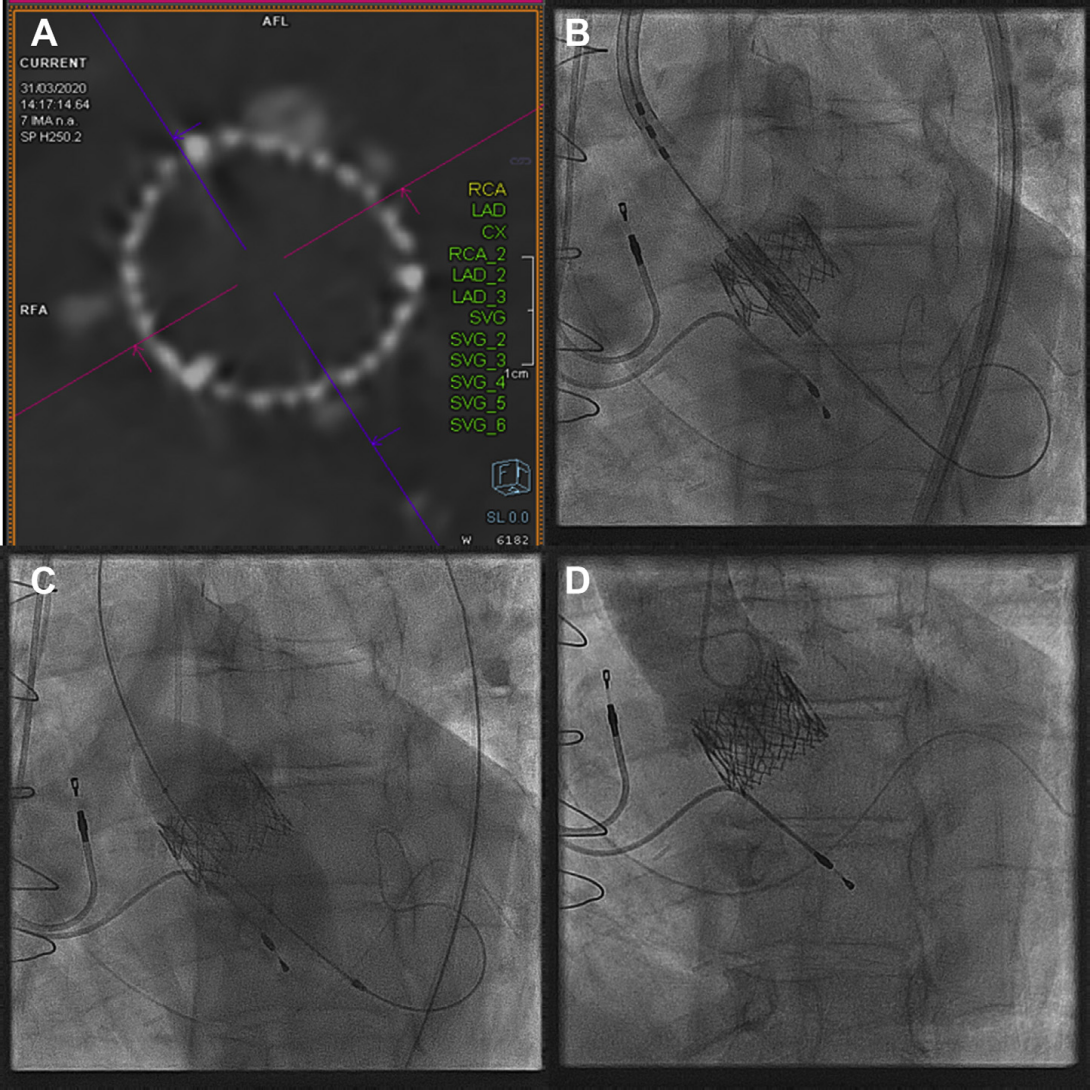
(A) Gated computed tomography aortogram (March 2020) showed complete absence of leaflet calcification of the Sapien XT (Edwards Lifesciences Corp, Irvine, CA) prosthesis (short axis left image, midframe level). (B) In April 2020 redo transcatheter aortic valve replacement was undertaken with a 26 mm Sapien 3 Ultra valve (Edwards Lifesciences Corp) via percutaneous transfemoral access. The top of the Sapien 3 Ultra was aligned with the top of the Sapien XT transcatheter heart valve. (C) Post dilatation was undertaken with a 26-mm Atlas Gold PTA Dilatation Catheter (Bard Peripheral Vascular, Inc, Tempe, AZ) balloon to 12 atm (nominal pressure 4 atm) to optimize expansion of the Sapien 3 and improve valve performance. (D) Root aortography after deployment and after optimization of the 26-mm Sapien 3 Ultra valve showed no aortic regurgitation.
